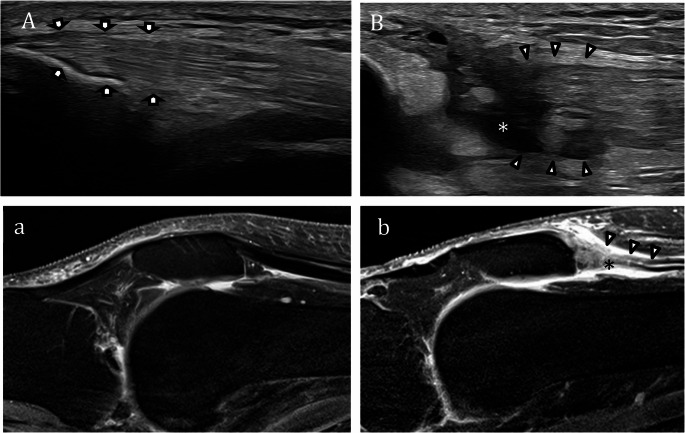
Complete tear of the quadriceps tendon A-a Longitudinal US and sagittal MRI PD fat-sat images demonstrate normal appearance of the quadriceps tendon (arrows) at its insertion onto the patella. B-b Longitudinal US image and sagittal fat saturation PD MRI image demonstrate a complete tear of the quadriceps tendon, with proximal retraction of the tendon stump (arrowheads). A surrounding hypoechoic (US) and hyperintense (MRI) fluid collection (*) is visible
